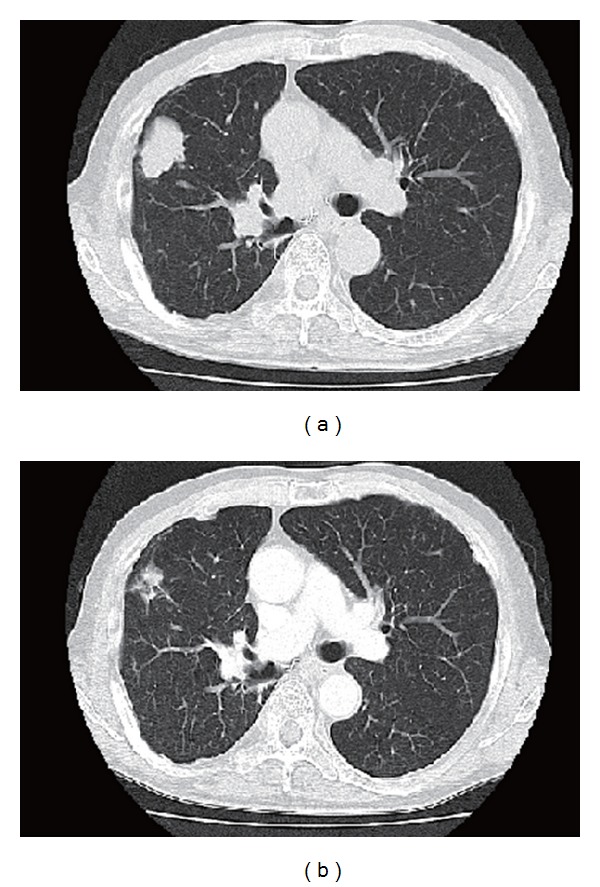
Chest CT of case 2 demonstrating tumor of the right upper lung (a). CT after the first cycle of the chemotherapy demonstrated remarkable response (b).
